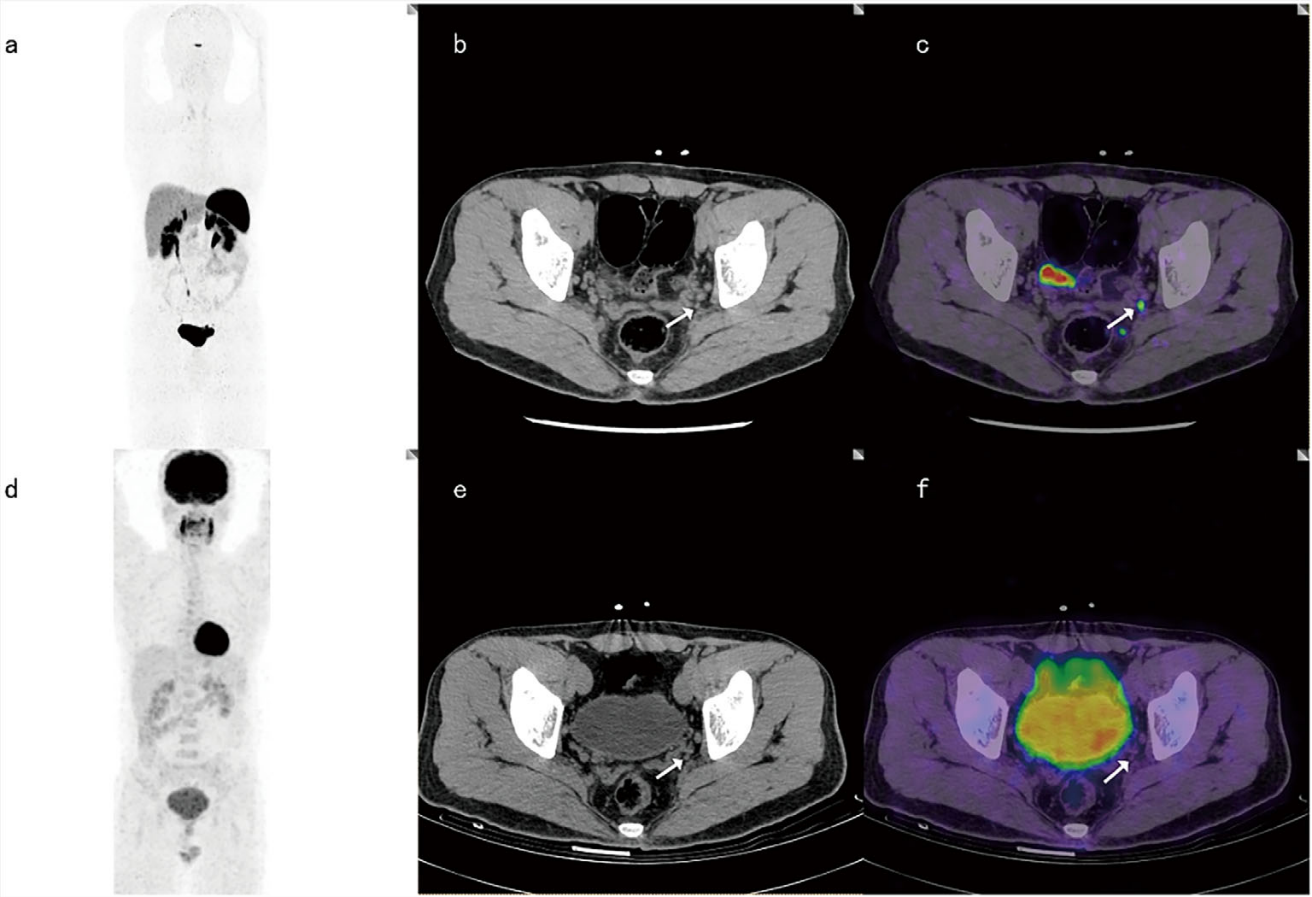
A 37-year-old male with LN metastasis adjacent to the left iliac blood vessel. The focal uptake of 68Ga-DOTANOC in LNs (as shown by the arrow) was obviously increased (A–C), while the focal uptake of 18F-FDG was similar to that in the background (D–F).
